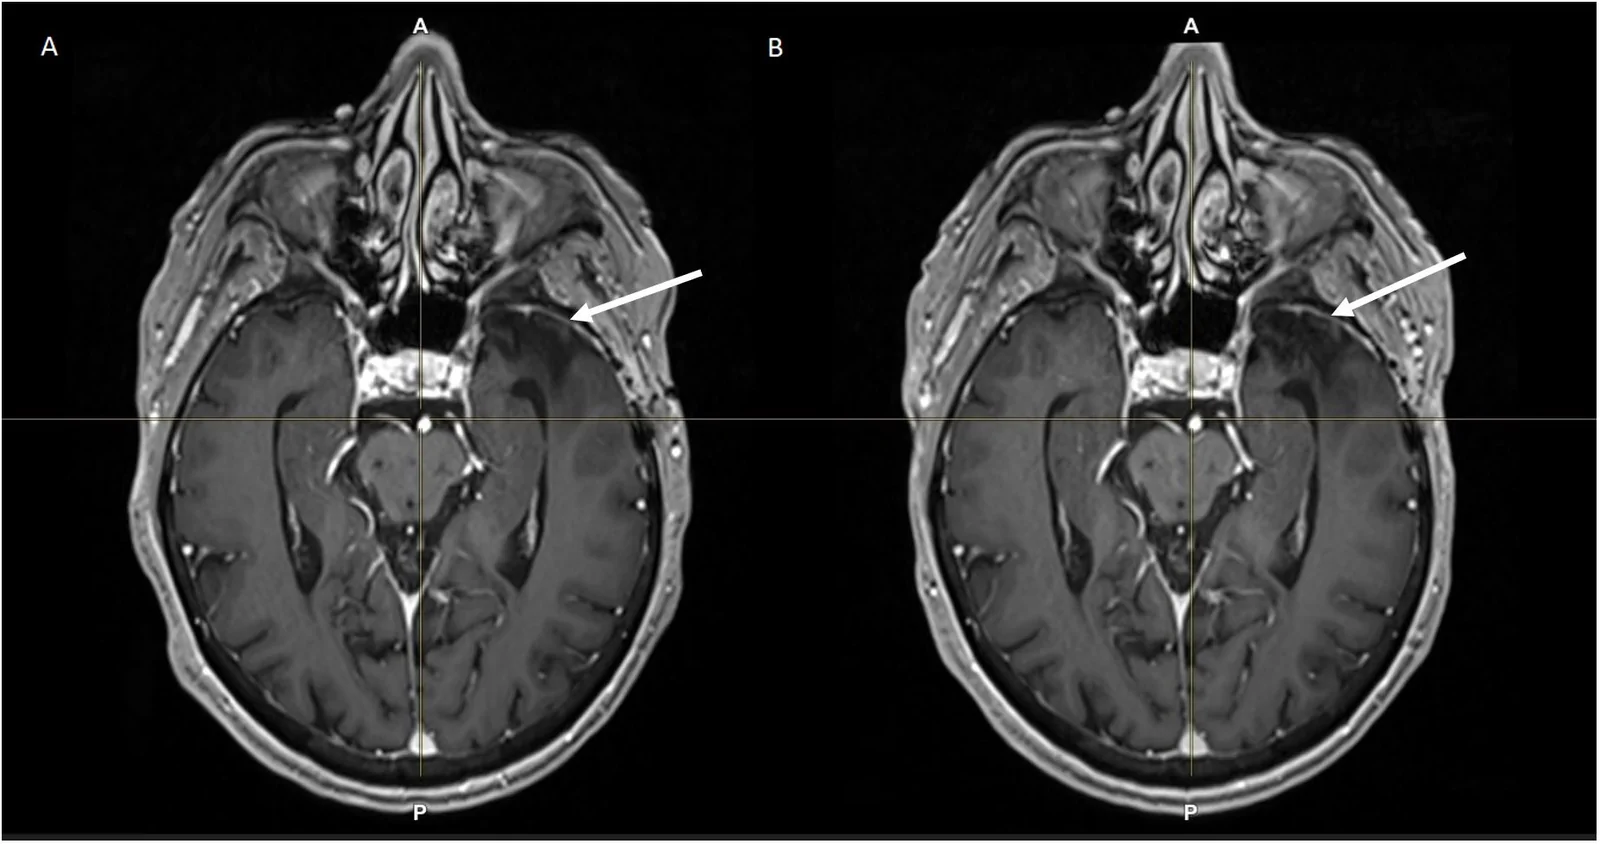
Follow-up contrast-enhanced T1-weighted MRI at 6 months (A) and 9 months (B) post-surgery showing post-operative changes without evidence of local recurrence.
